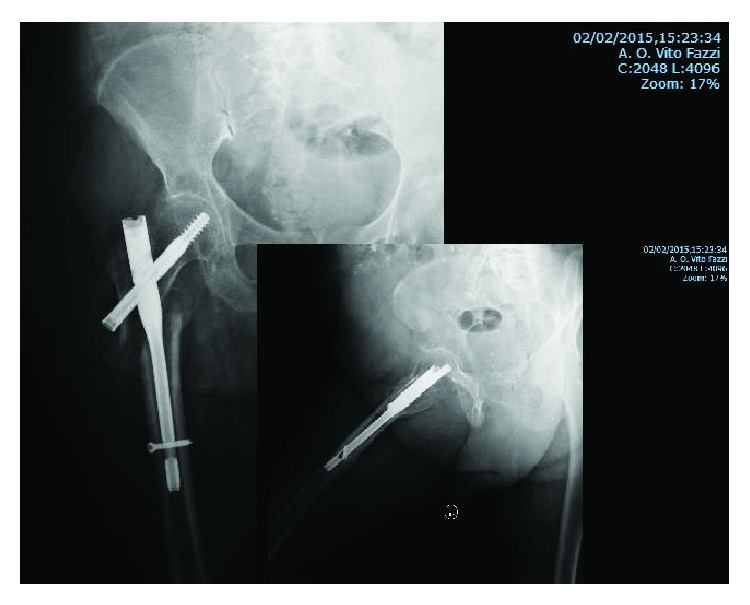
X-rays at 6 months of follow-up. Displacement of the fracture and aseptic nonunion.
